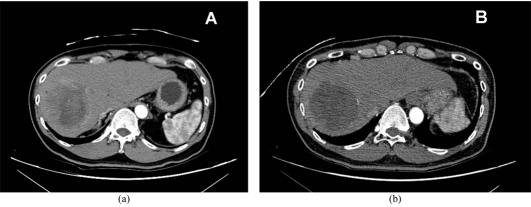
Multiphase CT in arterial phase showing a heterogenously enhancing lesion in the right lobe of liver (A). 1-month follow up CT in arterial phase shows tumour necrosis of most of the tumour with a small residual nodule at the periphery, in keeping with partial response (B).
